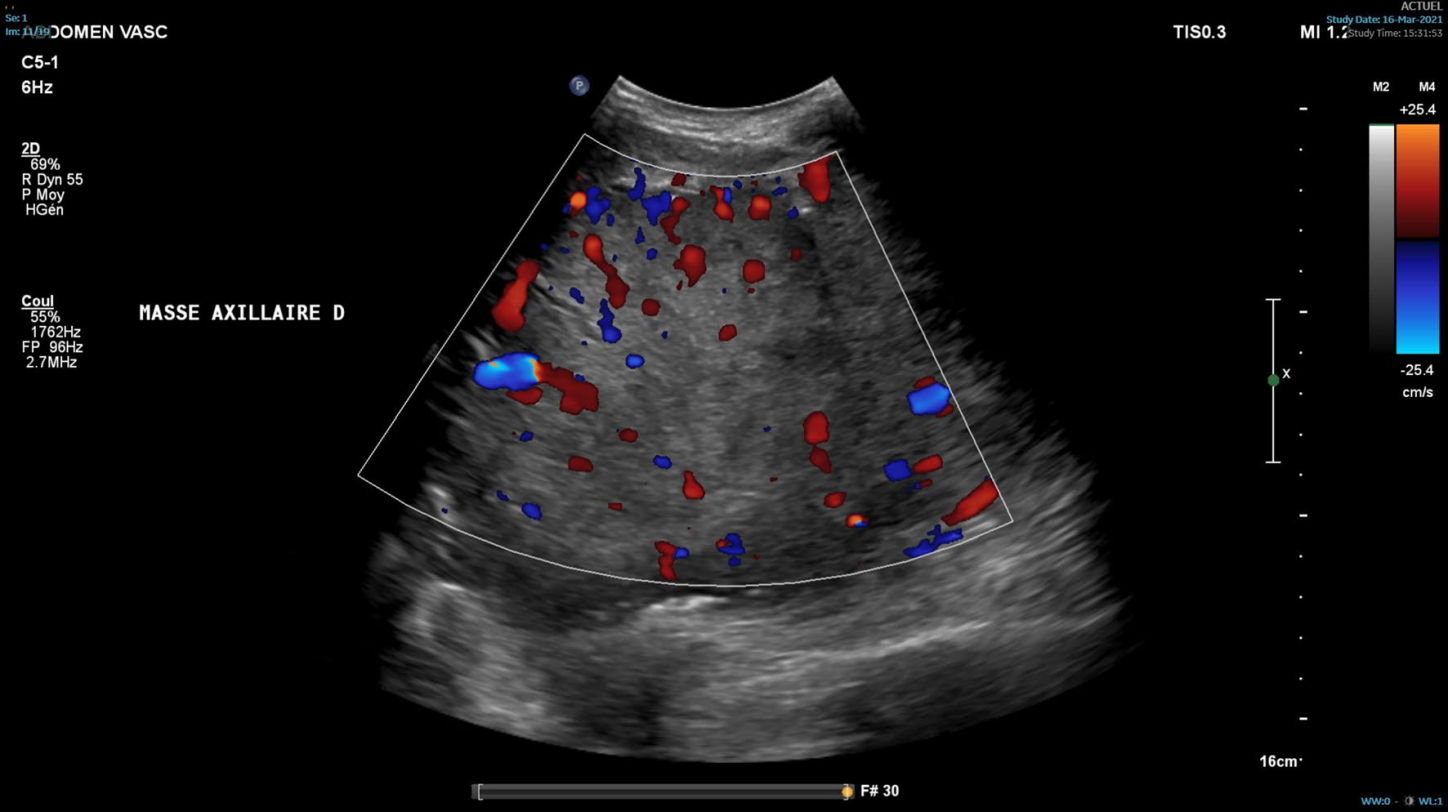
Doppler ultrasound image of numerous arteriovenous shunts within the right scapular mass
